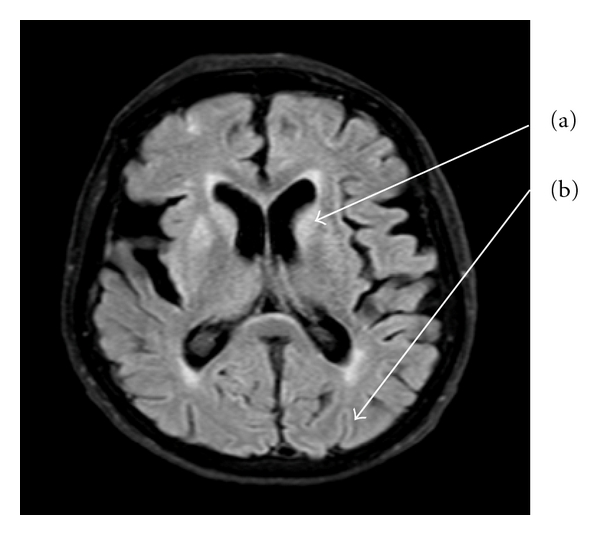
MRI scan demonstrating: (a) high signal in the caudate nucleus and (b) cortical ribboning.
